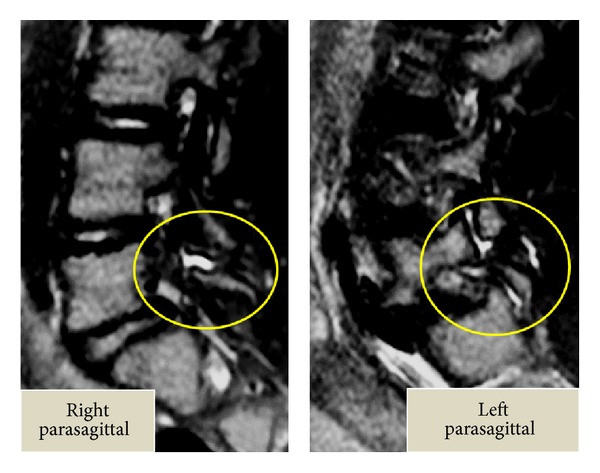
Sagittal short tau inversion recovery magnetic resonance imaging (STIR-MRI) of Case 1. Note the effusion in the pars defects and in the adjacent facet joints. These findings correspond with communicating synovitis.
